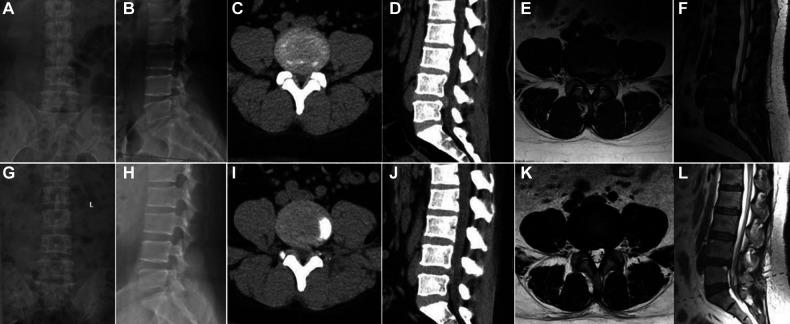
Pre- and postoperative x-ray, CT and MRI of PTED. (A,B) Preoperative x-ray. (C–F) Preoperative CT and MRI showing disc herniation. (G,H) Postoperative x-ray. (H,I) Postoperative CT and MRI showing the disc was removed.
